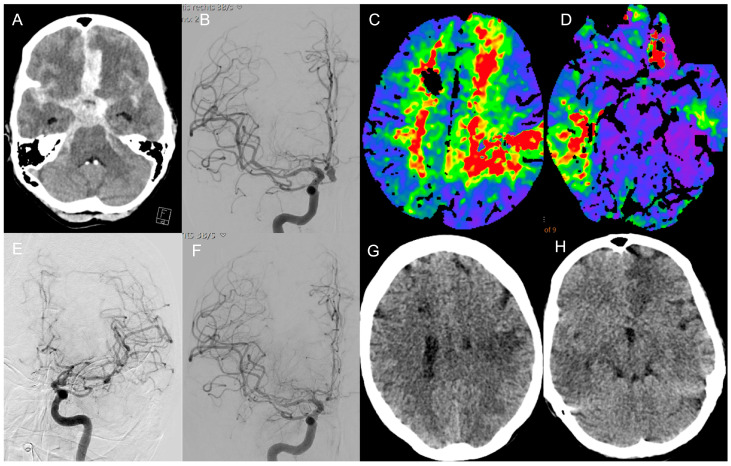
Representative case of early vasospasm. 56-year-old male patient with severe subarachnoid hemorrhage (A) due to an irregular ACoA aneurysm (B) treated with simple coiling. Three days later, the patient presented with elevated flow velocities in TCD. Subsequent CT perfusion demonstrated bilateral frontoparietal perfusion delay (C) and right temporal perfusion delay (D). Digital subtraction angiography (DSA) revealed moderate vasospasm of both the middle cerebral and anterior cerebral arteries (E,F). The patient underwent five sessions of intra-arterial spasmolysis in both territories of the internal carotid artery. The patient developed secondary infarctions in both MCA territories (G,H) as well as in the left ACA territory (H) and ultimately died during hospitalization. Note: focal parenchymal changes along the EVD catheter tract may be present and should not be interpreted as vasospasm-associated territorial ischemia.
